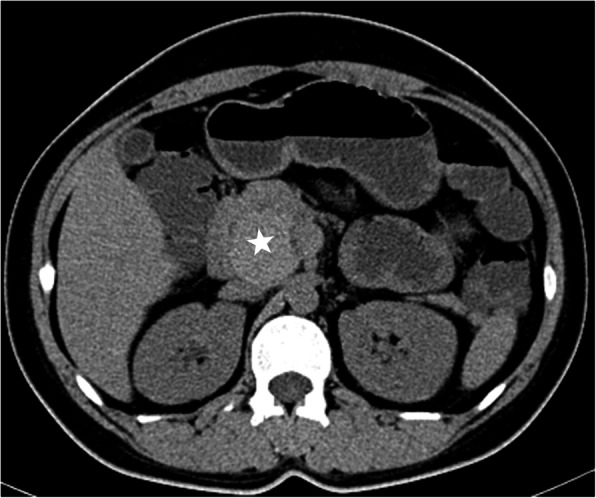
Tumor was demonstrated a lobulated, partly ill-defined lesion (star) with predominantly homogeneous hyperattenuation (mean CT value = 60HU) located on the posterior of head of pancreas on non contrast CT
